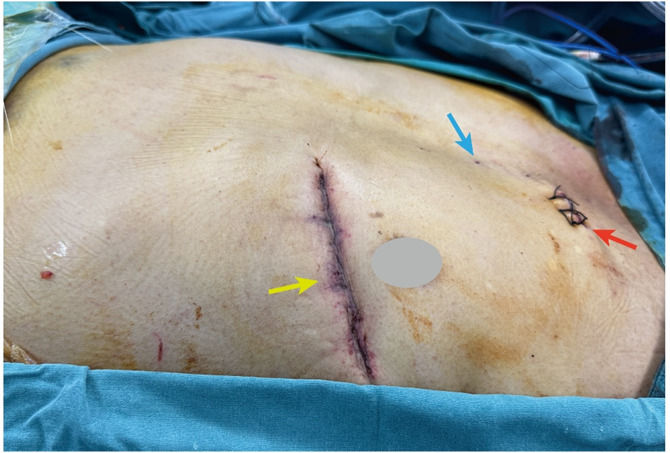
Overview of incisions. Main incision of left fifth intercostal thoracotomy (yellow arrow) and left second intercostal space for side-biting clamp insertion (red arrow) and skin puncture points of intercostal parasternal spaces (blue arrow)
